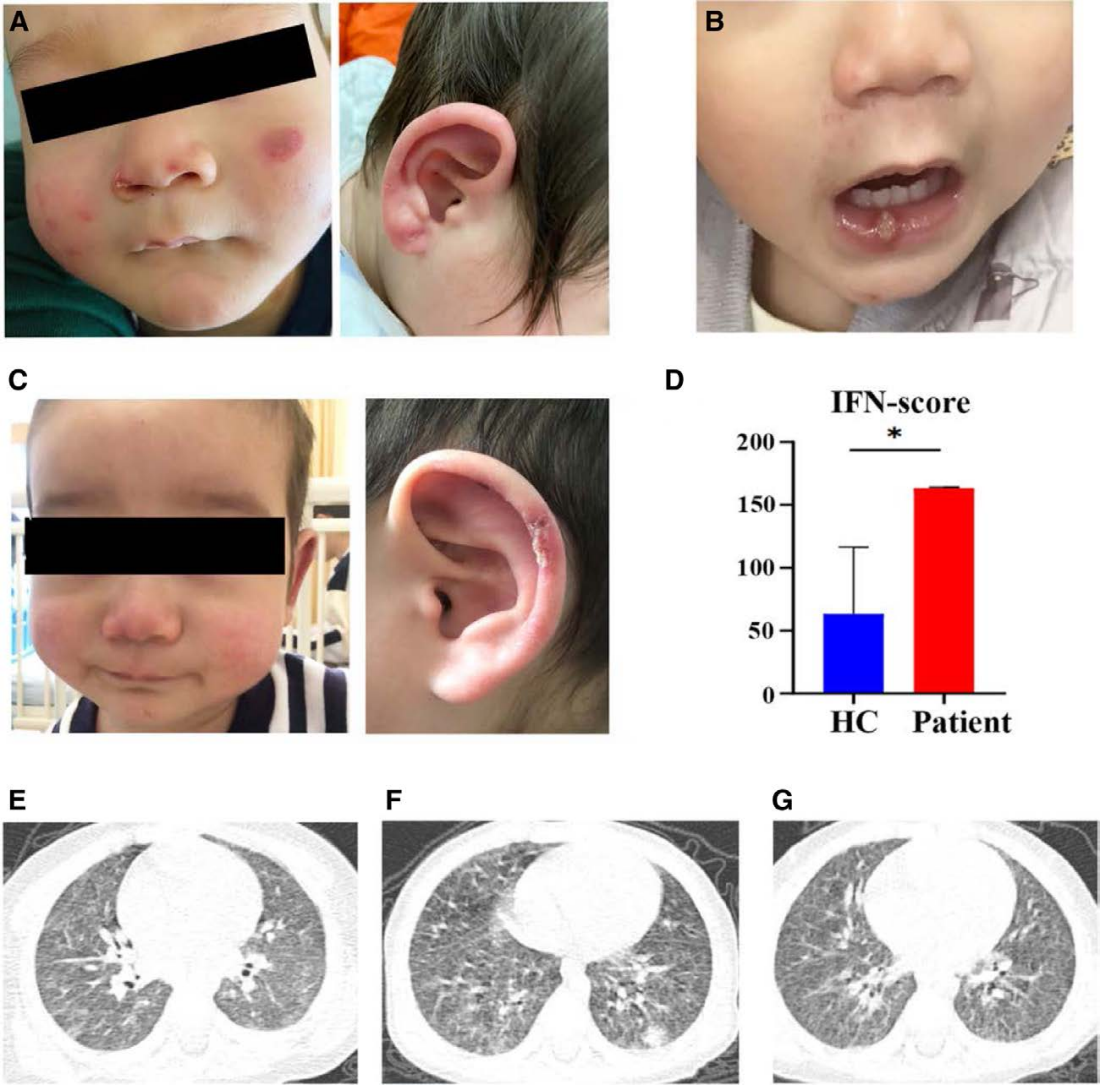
Clinical features of the patient. (A) Photograph of the facial rashes were present on the cheeks, nose tip, and auricles on both sides. (B) Photograph of patient with oral ulcer. (C) Photograph of the facial rashes after treatment of tofacitinib. (D) The expression of IFN regulated genes in health control (HC) and patient. (E–G) Chest CT of patient. CT = computed tomography, IFN = interferon.
